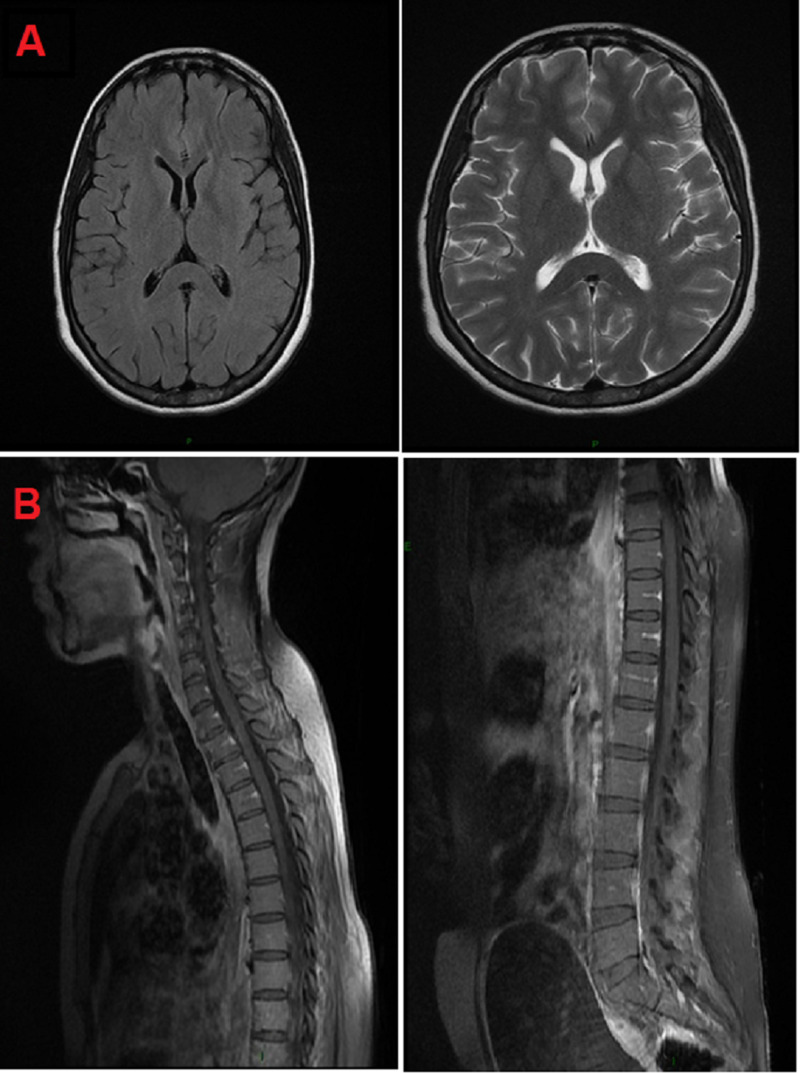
Encephalic (A) and medullary (B) MRI of our patient showing no abnormalities
